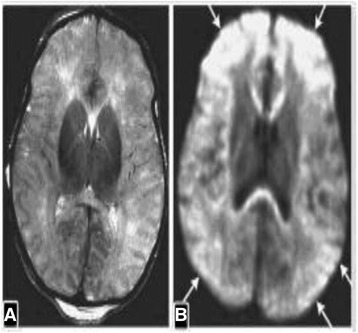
MRI scans of severe Partial Prolonged Asphyxia: A 4-day-old infant owing to tight cord around neck. A) Axial T2- weighted imaging shows abnormal cortical and subcortical bilateral cerebral increased T2 signal intensity. B) Axial diffusion weighted imaging shows extensive gray and white matter injury (arrows) of both cerebral hemispheres – From KN V: Cerebral Palsy and Early Stimulation. New Delhi, India: Jaypee Brothers. Medical Publishers (P) Ltd., 2014, with permission
